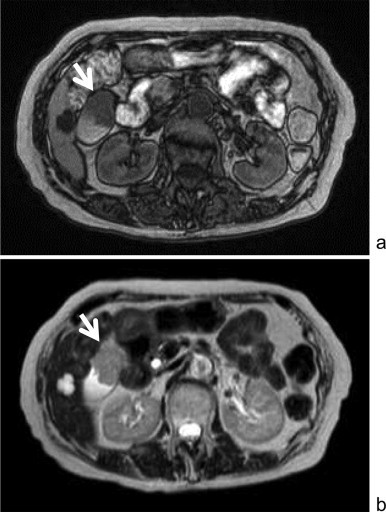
MRI demonstrated that the gallbladder tumor (arrow) showed low signal intensity on T1-weighted images and slightly high on T2-weighted images. (a) T1-weighted images. (b) T2-weighted images.
